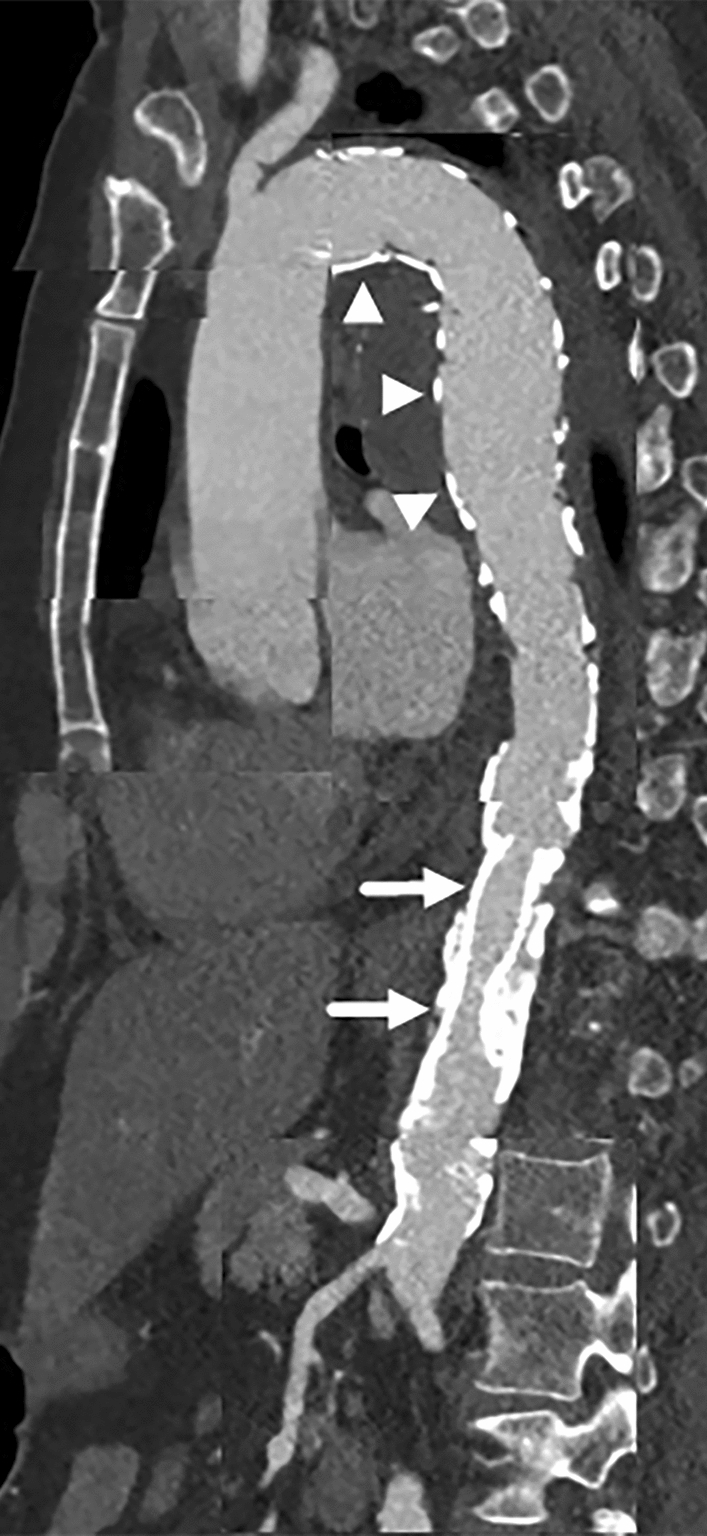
CT-angiography 6 days after intervention (composed reformatted images): Complete occlusion of the false aneurysm by the aortic endoprosthesis (arrowheads). Patent BeGraft® (arrows)
